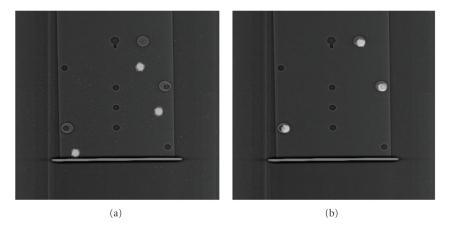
Accuracy of lateral distortion compensation algorithm for planar scintigram-CT topogram registration. Fused images of the original (uncompensated) scintigram and CT topogram of three button sources (a), and stretched (compensated) scintigram and CT topogram (b). The vertical shift in the original fused images is due to a registration offset in the table travel direction of the scanner equal to the distance between the edge of the field of view of the gamma camera detector and the edge of the image matrix. This offset is accounted for in the compensation algorithm so the scintigram and topogram accurately register in the longitudinal direction of the scanner. On the right, the scintigram is compensated to correct the lateral position of the right-most button source. The residual error in lateral position of a given button source after compensation was within ±3.44 mm.
